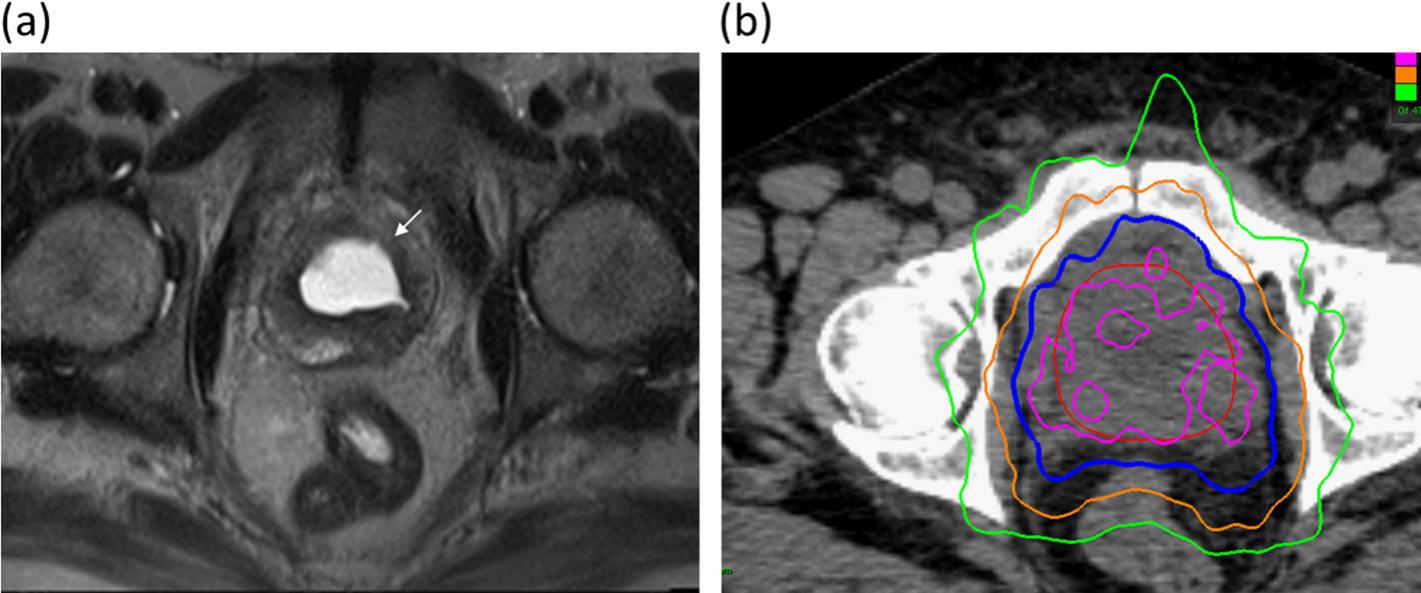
Treatment plan. (a) Axial T2-weighted MR image demonstrating central simple prostatectomy defect (arrow). (b) Treatment planning axial CT images demonstrating the prostate (red line). Isodose lines shown as follows: 115% of the prescription dose, pink line; 100% of the prescription dose, blue line: 75% of the prescription dose, orange line; and 50% of the prescription dose, green line.
